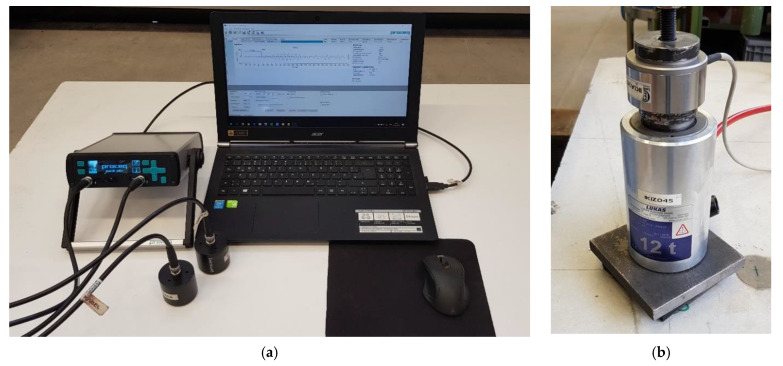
Overview of ultrasonic measurement configurations: (a) used ultrasound device Pundit Lab+; (b) test setup for load application.
